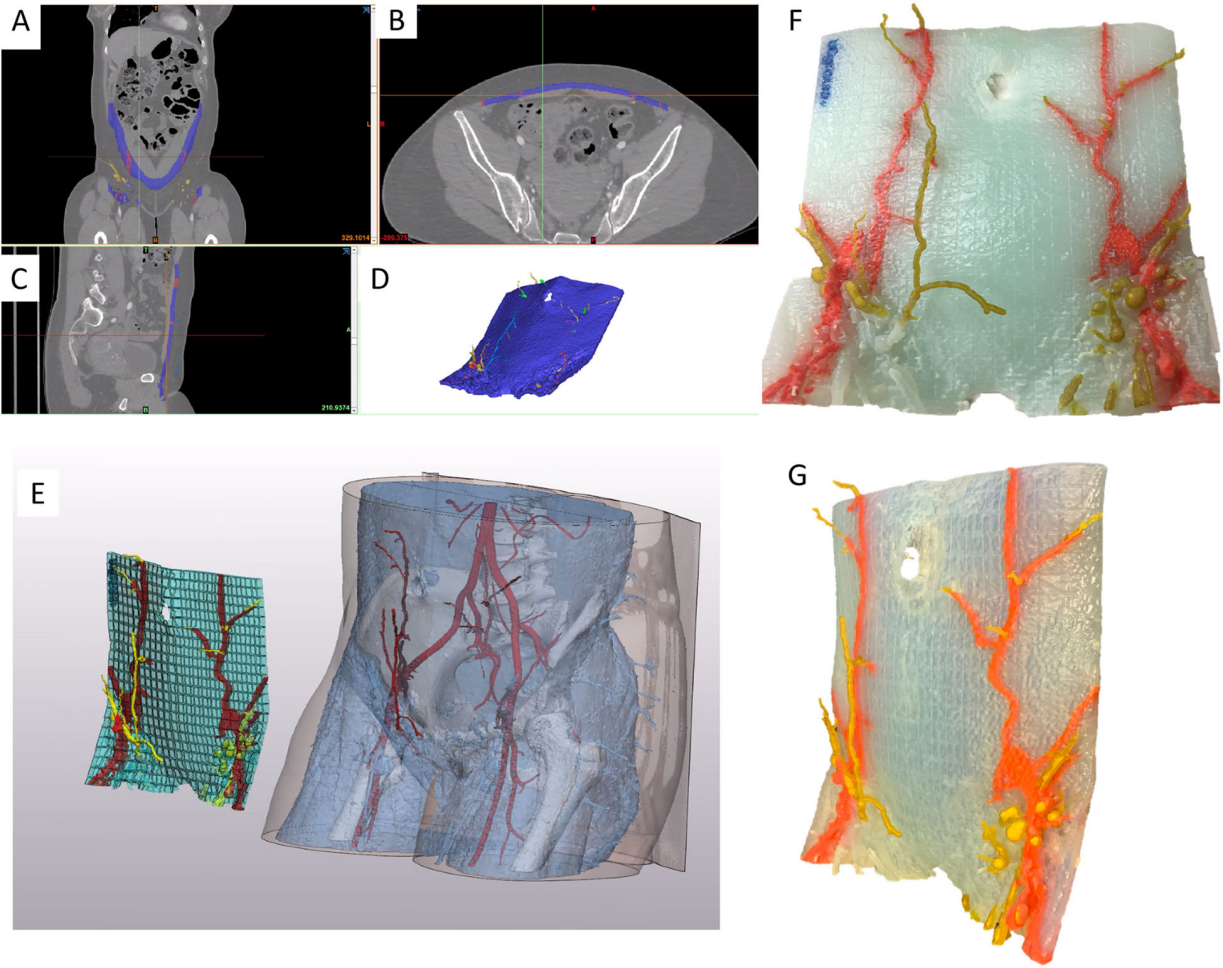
a Coronal, b axial, and c Sagittal images from a preoperative CTA are used to create a 3D model depicting the deep inferior epigastric vascular tree. A segmentation software (MIMICS; Materialise, Belgium) is used isolate vessels of interest and the abdominal muscle inorder to highlight the intramuscular course of these vessels (d, e). The virtual model is subsequently printed using the Stratasys Connex J735 3D printer (Eden Prairie, Minn) and is shown in the coronal (f) and (g) sagittal projection. This model can be used intraoperatively to guide dissection of vessels
